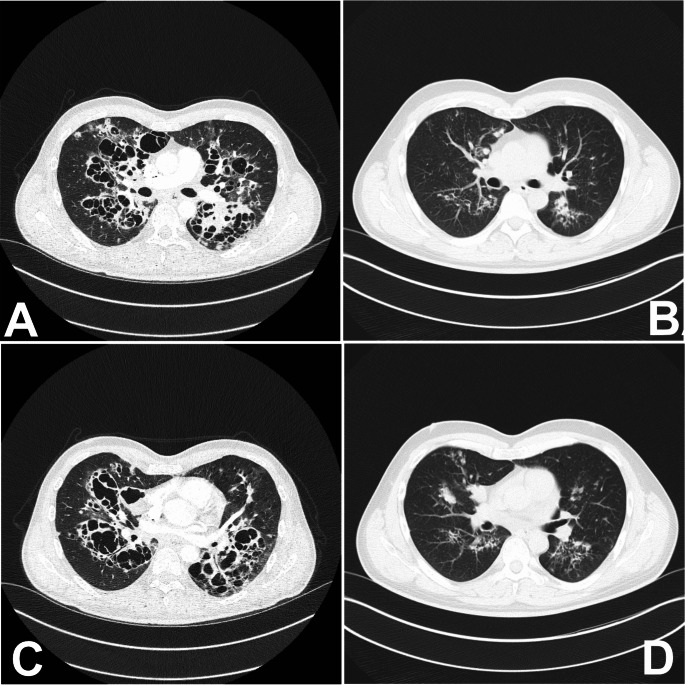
Figure 1 A&C: Radiological image (CT scan) at presentation Figure 1 B&D: Radiological image after three months
